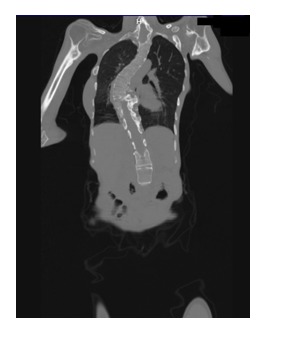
CT aspect. Dextro-converse scoliosis; bone thorax deformation. Pulmonary fibrosis.
